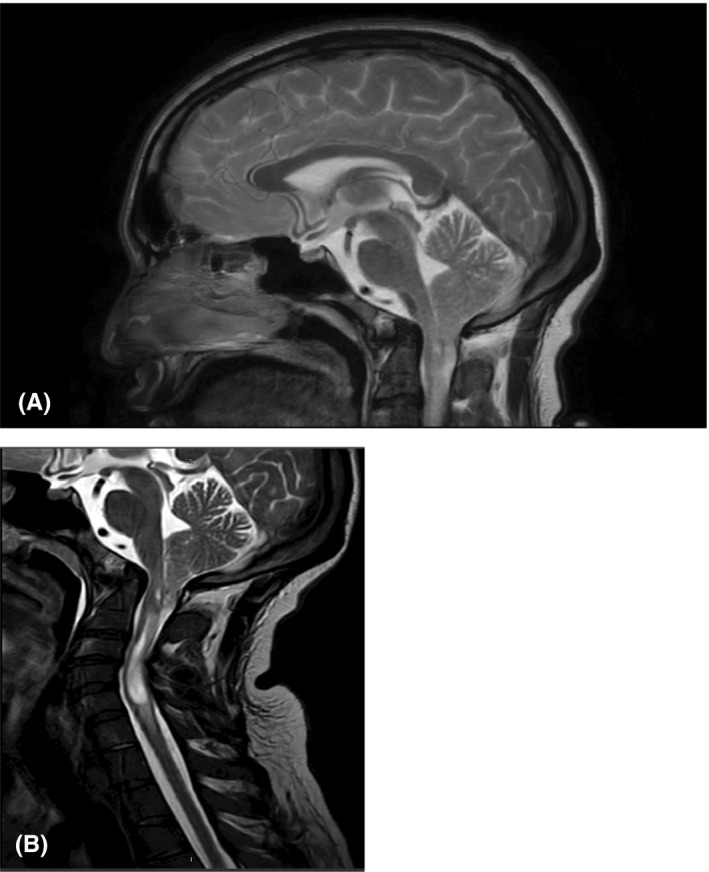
A and B, Sagittal magnetic resonance image of the brain. Note the cervico‐medullary junction compression
